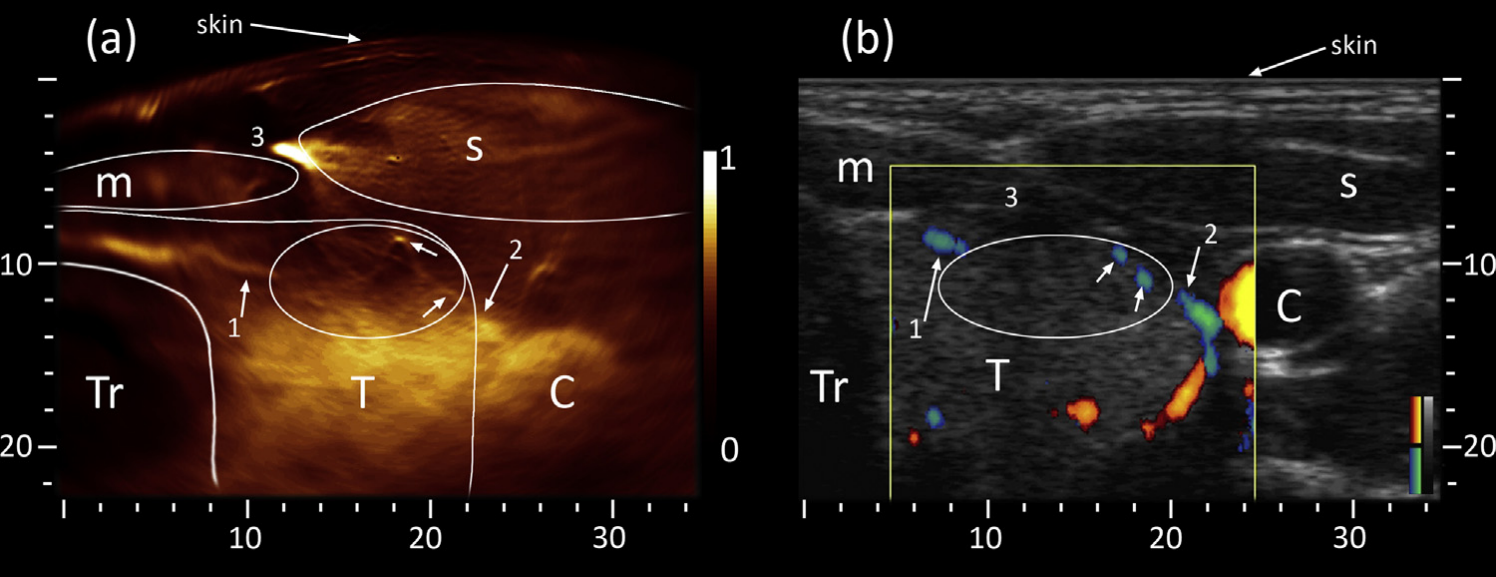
Cross-section of right thyroid lobe of the second volunteer. (a) To limit the influence of strong absorbers (large vein marked 3) on available contrast the optoacoustic image was range limited with respect to high intensity values before leveling and normalization from 0 to 1. Besides larger features (e.g. muscles, thyroid) optoacoustic imaging allows identification of prominent vascularization [1], [2] and a fine vascular network (oval highlight) stretching inside the imaging plane and perpendicular to it (fine dots). (b) The corresponding ultrasound image confirms features detected in (a), whereby grayscale represents the pulse-echo and color the Directional Power Doppler signal (red/blue colormaps indicating opposite flow directions). C: Carotid, T: Thyroid, Tr: Trachea, s: sternocleidomastoid muscle, m: infrahyoid muscle; axes in mm.
